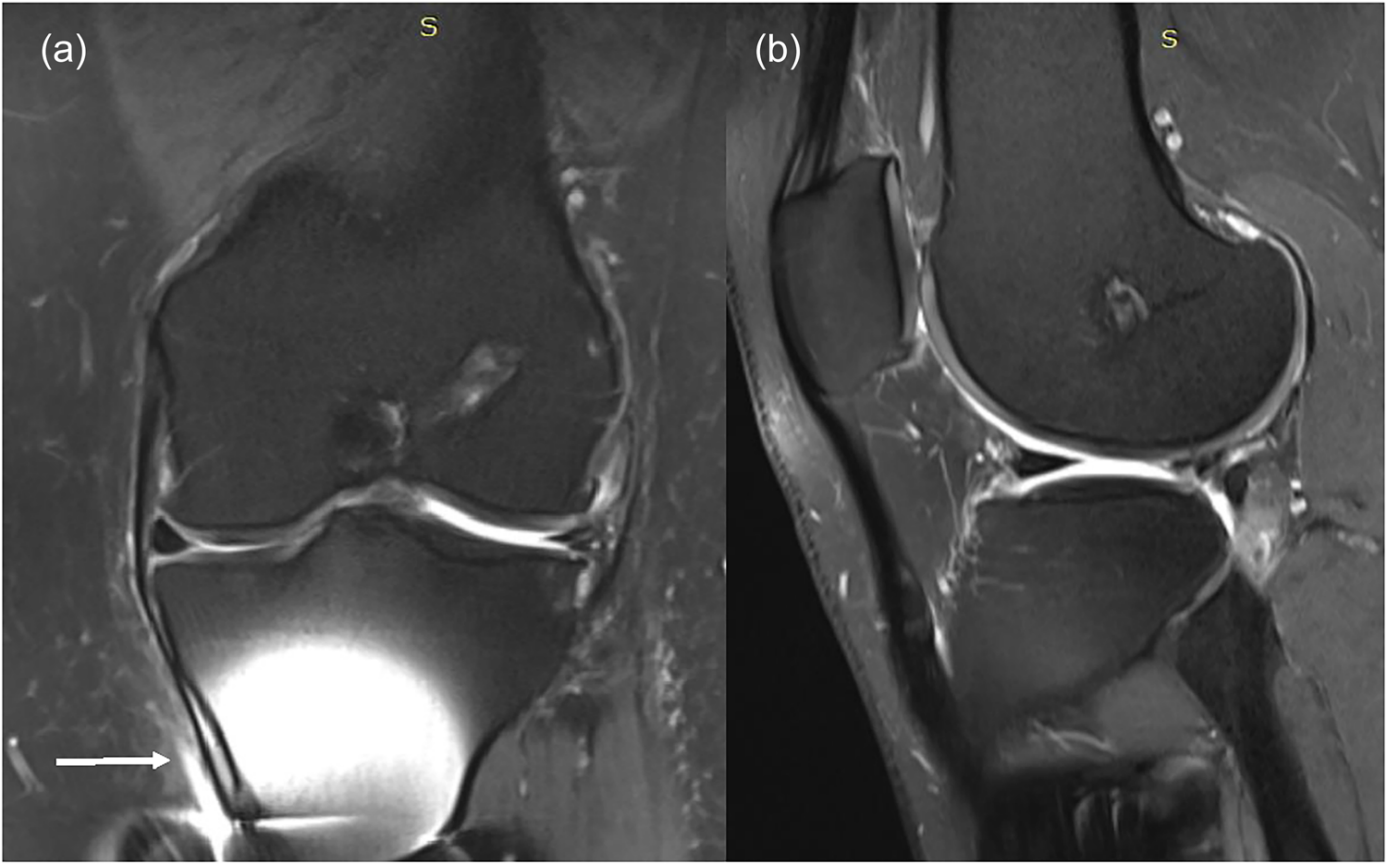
MRI of the left knee (a: coronal view—fat saturation, b: sagittal view—fat saturation) 11 years post‐operation on the lateral meniscus shows a maintained implant, though smaller in size, with partial extrusion and a tear. The white arrow indicates bone oedema, likely an artefact from ACL reconstruction. ACL, anterior cruciate ligament; MRI, magnetic resonance imaging.
